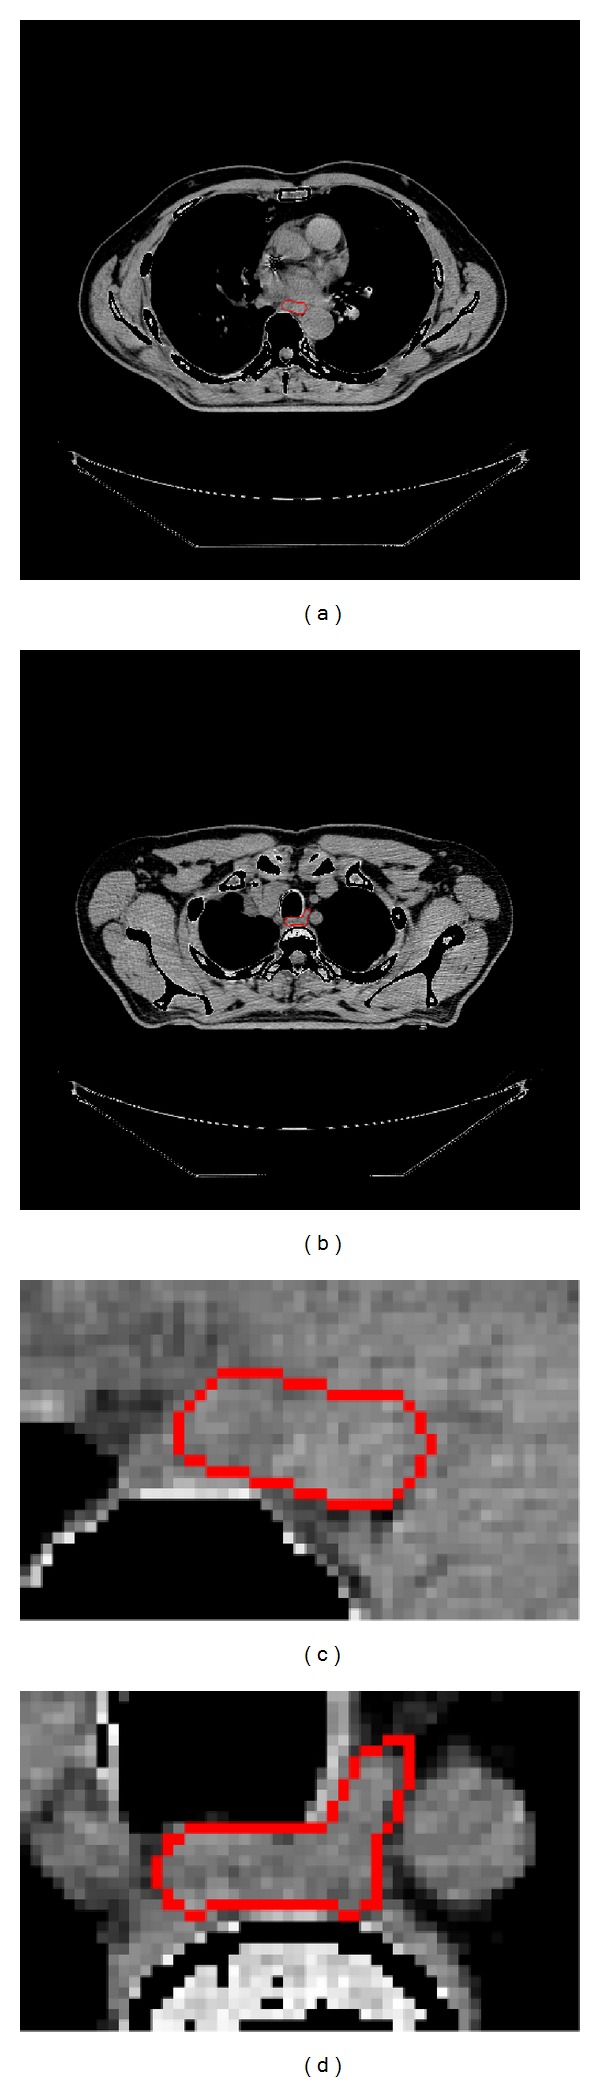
CT scan ((a), (b)) and cropped ROI from CT scan ((c), (d)) with esophagus manually segmented in red. Note the variable shape of the esophagus and how its grey levels are similar to surrounding tissues.
